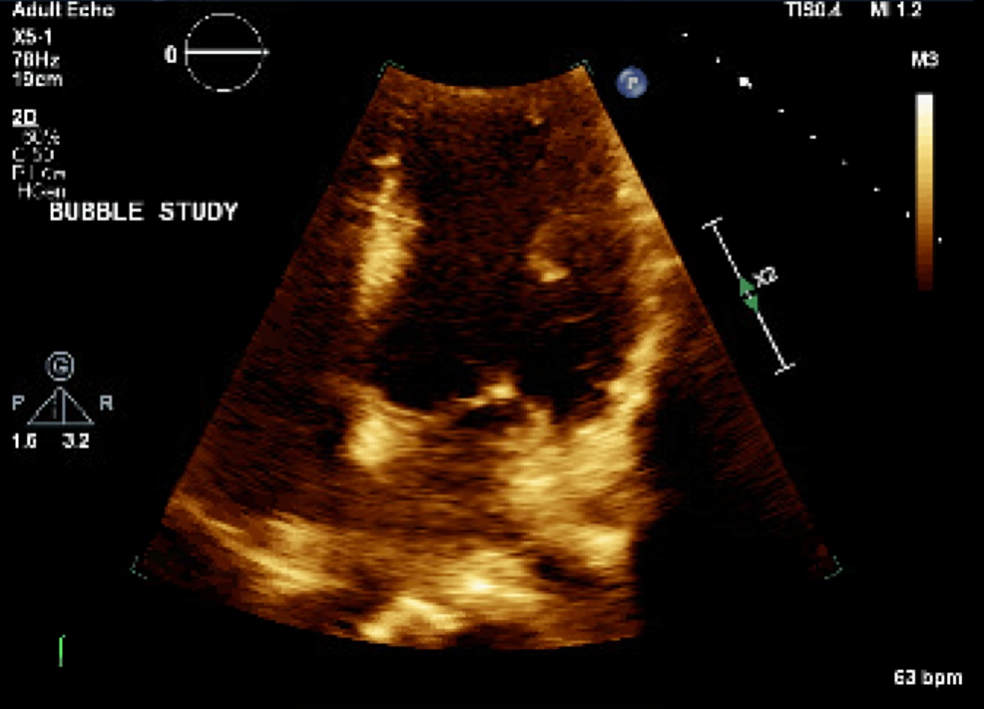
Transthoracic echocardiogram with agitated saline showed evidence of a PFO, and transesophageal echocardiogram with a bubble study confirmed the presence of a PFO measuring 2 to 3 mm. PFO, patent foramen ovale
